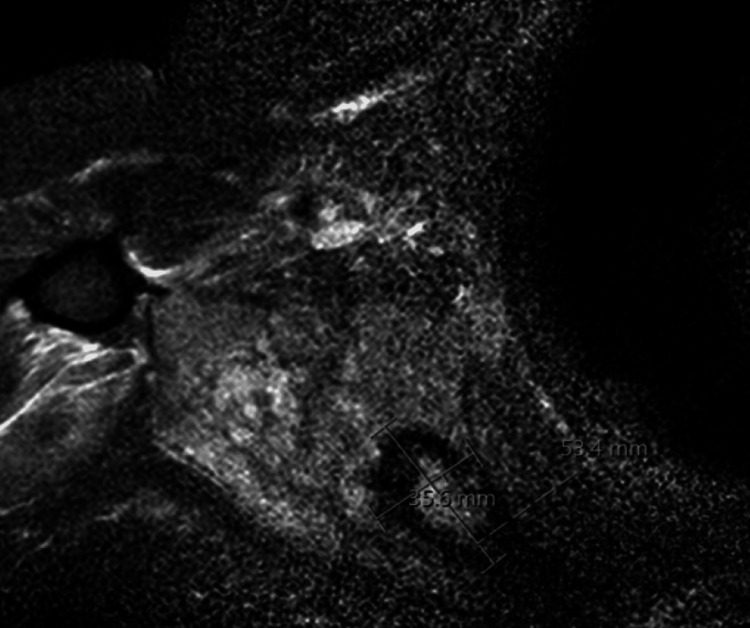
Post-treatment MRI Post-treatment T2 axial MRI showing evidence of edema and enhancement in previously treated region
